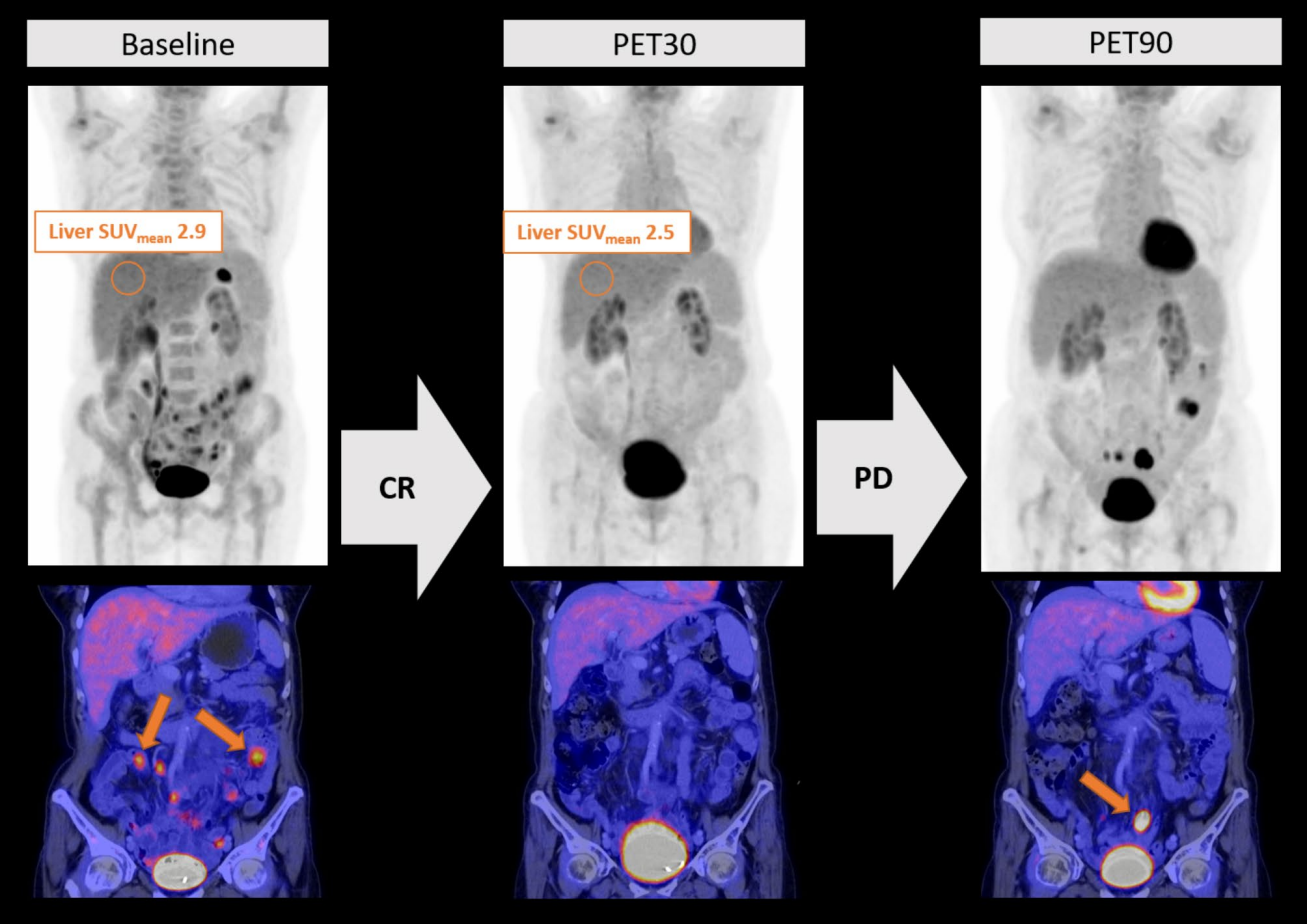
Decrease in Liver-SUV mean is associated with early progression after CAR-T. Representative PET/CT images of in relapse depicting lymphoma at baseline (first column) with multiple FDG-avid intestinal lesions (orange arrows in lower row). PET30 images (second column) demonstrate early metabolic complete remission. Representative measurements of the Liver-SUV_mean_ at Baseline and PET30 are shown in the upper row. PET90 images (third column) depict multiple new intestinal FDG-avid lesions of which the most prominent is marked with an orange arrow in the lower row. CD19-CAR-T cell therapy (CAR-T), Fluor-18-Deoxyglucose (FDG), PET/CT scan at day 30 (PET30), PET/CT scan at day 90 (PET90)
